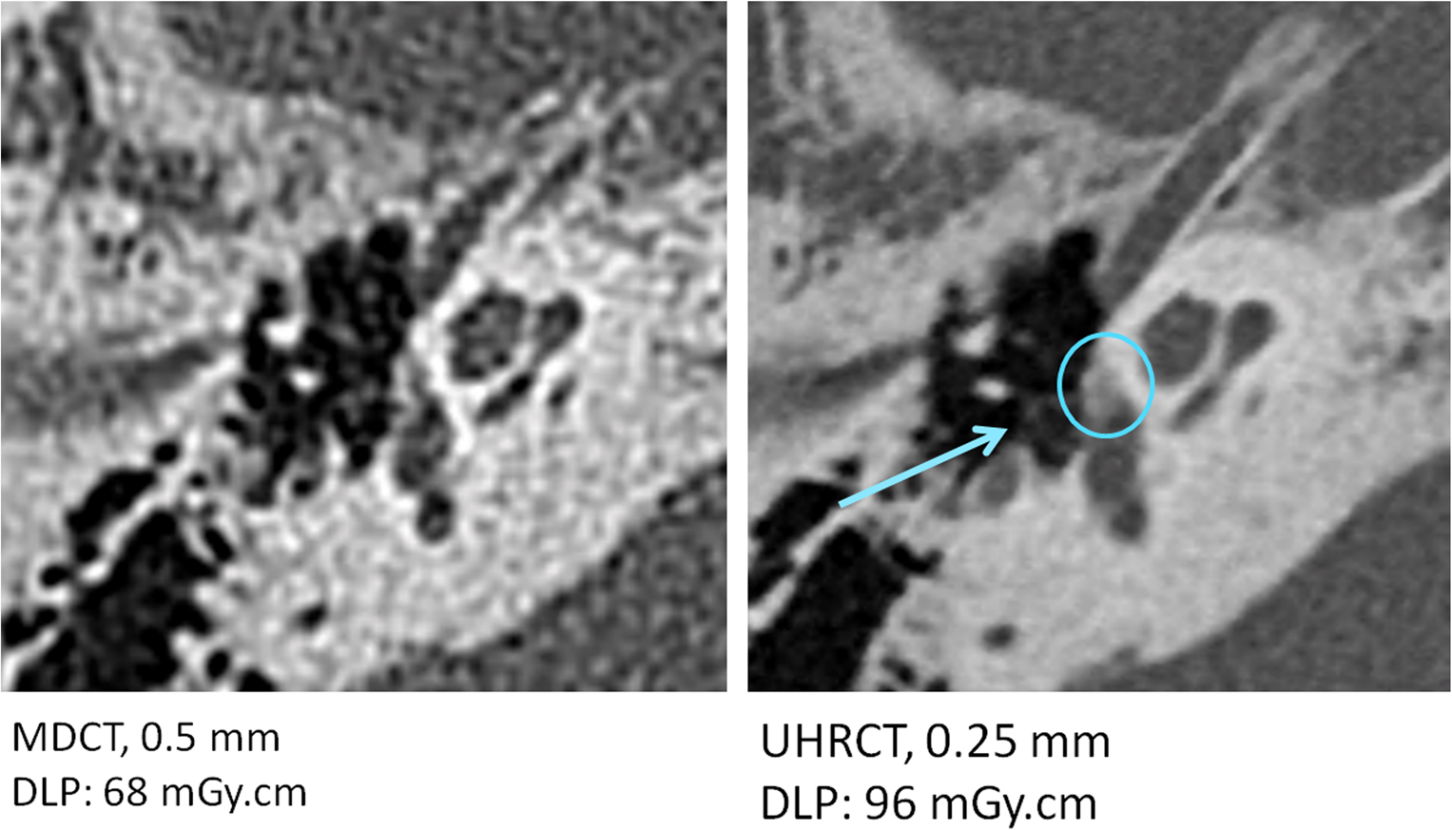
Two different right ear bone kernel CT scans of fenestral otosclerosis as a subtle, focal area with lucency in front of the stapedial footplate (circle). (Left) MDCT and (right) UHRCT in HR mode, both using hybrid-iterative reconstruction. The UHRCT scan contains less noise and enables better delineation of the stapes (arrow), the trabeculae of the medullary bone of the mastoid, the bony otic capsule and the focal lucency (circle) in this capsule representing the disease
